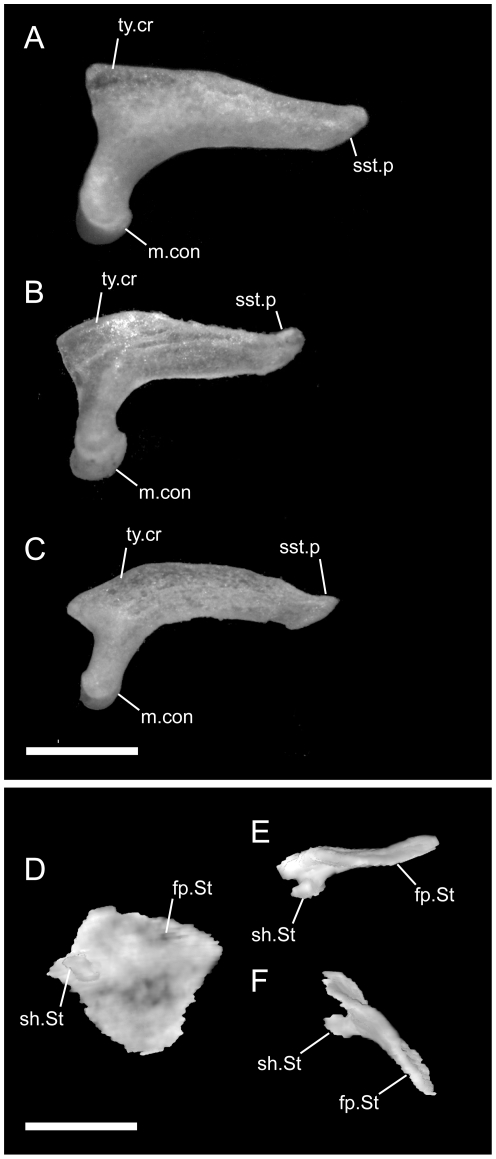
Disarticulated quadrates and stapes. Anterior is to the left unless noted; scale bars = 0.5 mm. A from *U. woodmasoni* (TMM M-10001); B from *U. melanogaster* (TMM M-10045); C from *B. rhodogaster* (TMM M-10027); and D–F from CT scans of *U. woodmasoni* (TMM M-10006). Left quadrates in lateral view (A–C), and right stapes in ventrolateral (K, anterior to the right), dorsal (L, anterior to the right), and anterolateral (M, lateral to the left) views. fp.St = stapedial footplate; m.con = mandibular condyle of quadrate; sh.St = stapedial shaft; sst.p = suprastapedial process (caudal process) of quadrate; ty.cr = tympanic crest of quadrate.
